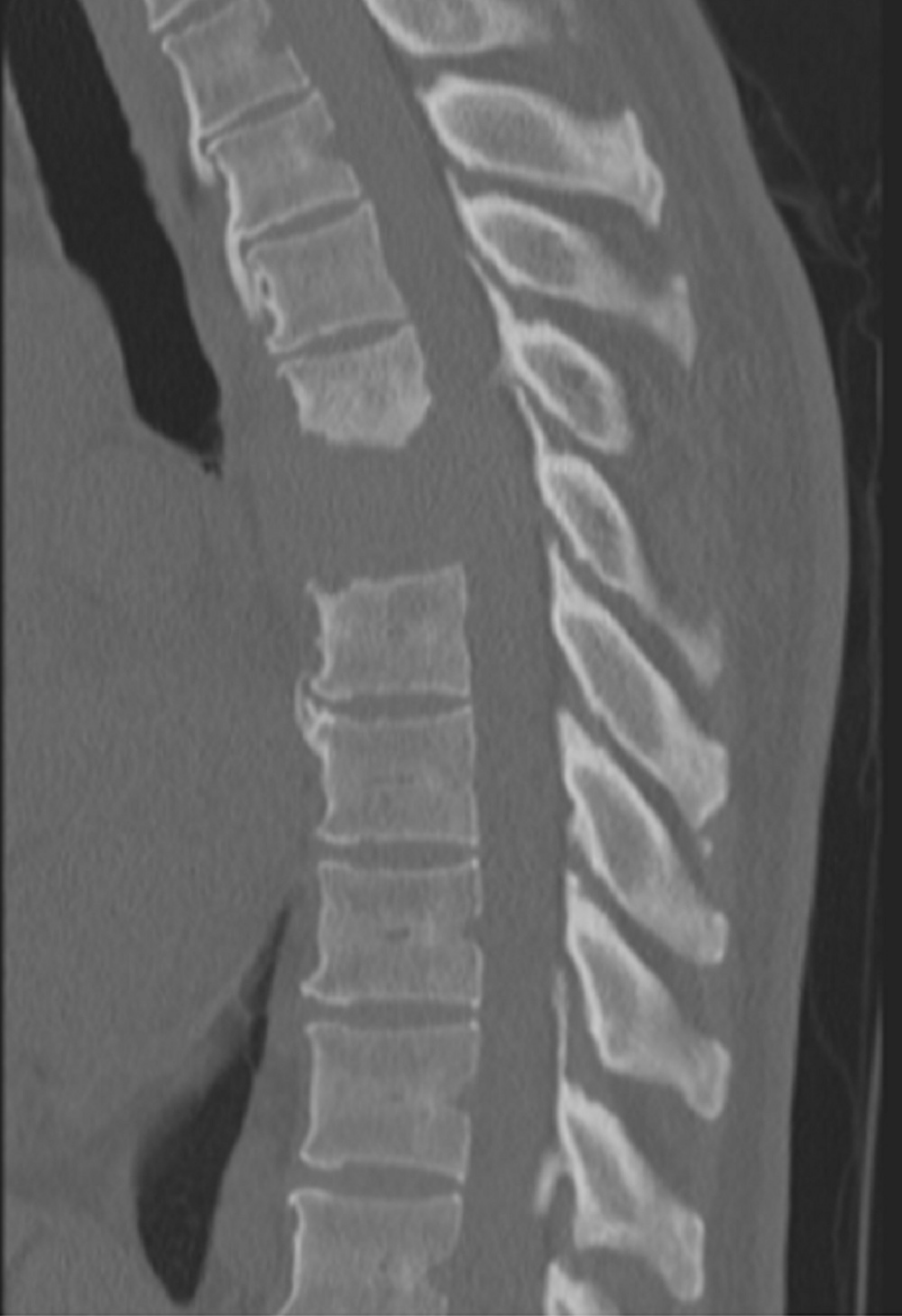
Sagittal CT of the thoracic spine Sagittal computed tomography (CT) of the thoracic spine demonstrating a lytic bone lesion primarily involving the T7 vertebra with some extension cranially into T6 and mild caudal extension into the superior endplate of T8.
